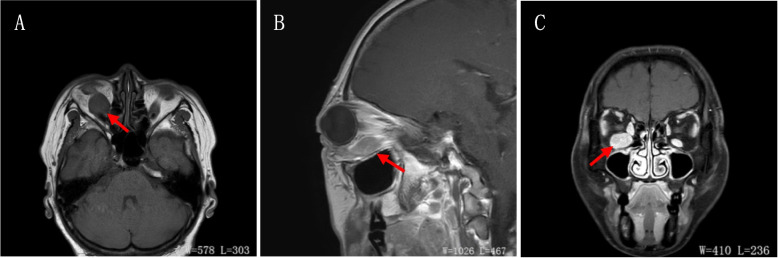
Magnetic resonance imaging of the right inferior rectus muscle enlargement (red arrow) in 3 different images: A T1-weighted axial image; B T1-weighted sagittal image; C Post-contrast T1-weighted coronal image
